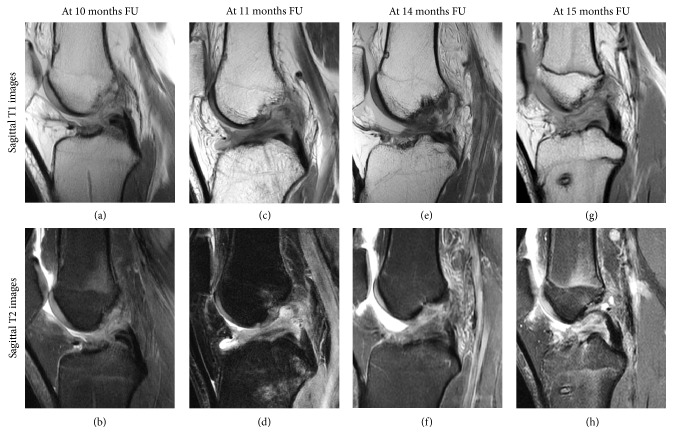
Sagittal T1 (upper row) and T2 (lower row) MR images of four patients are shown with a rerupture of the repaired ligament.
